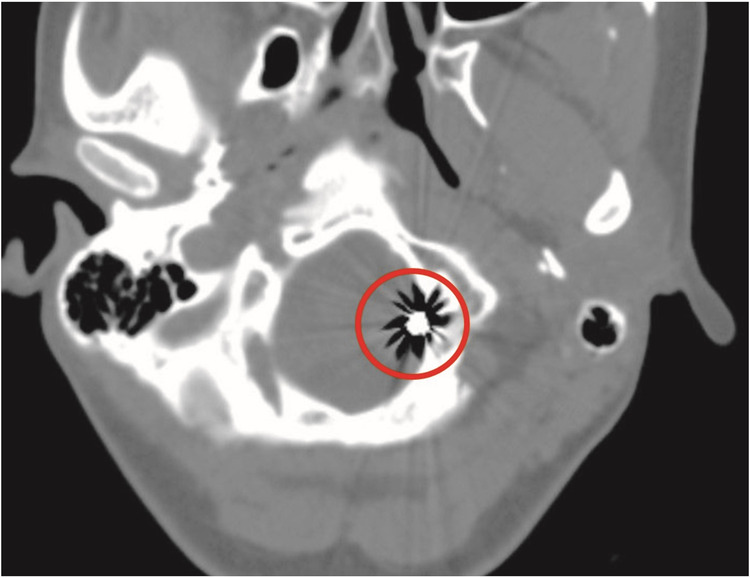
Postoperative non-contrast CT head demonstrating left posterior inferior cerebellar artery (PICA) aneurysm filled with coils (red circle).
